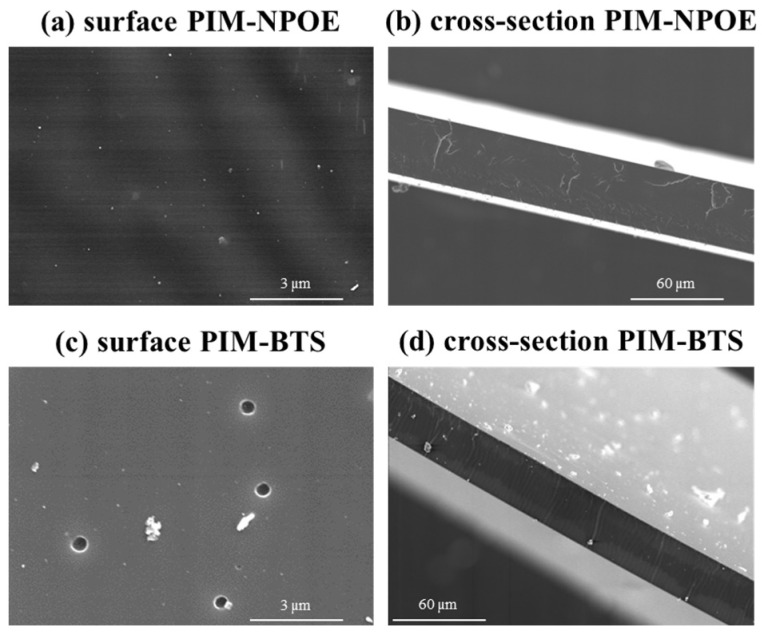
SEM images of PVC-based PIMs (50% CTA, 30% TOMATS, and 20% plasticizer (a,b) NPOE or (c,d) BTS).
